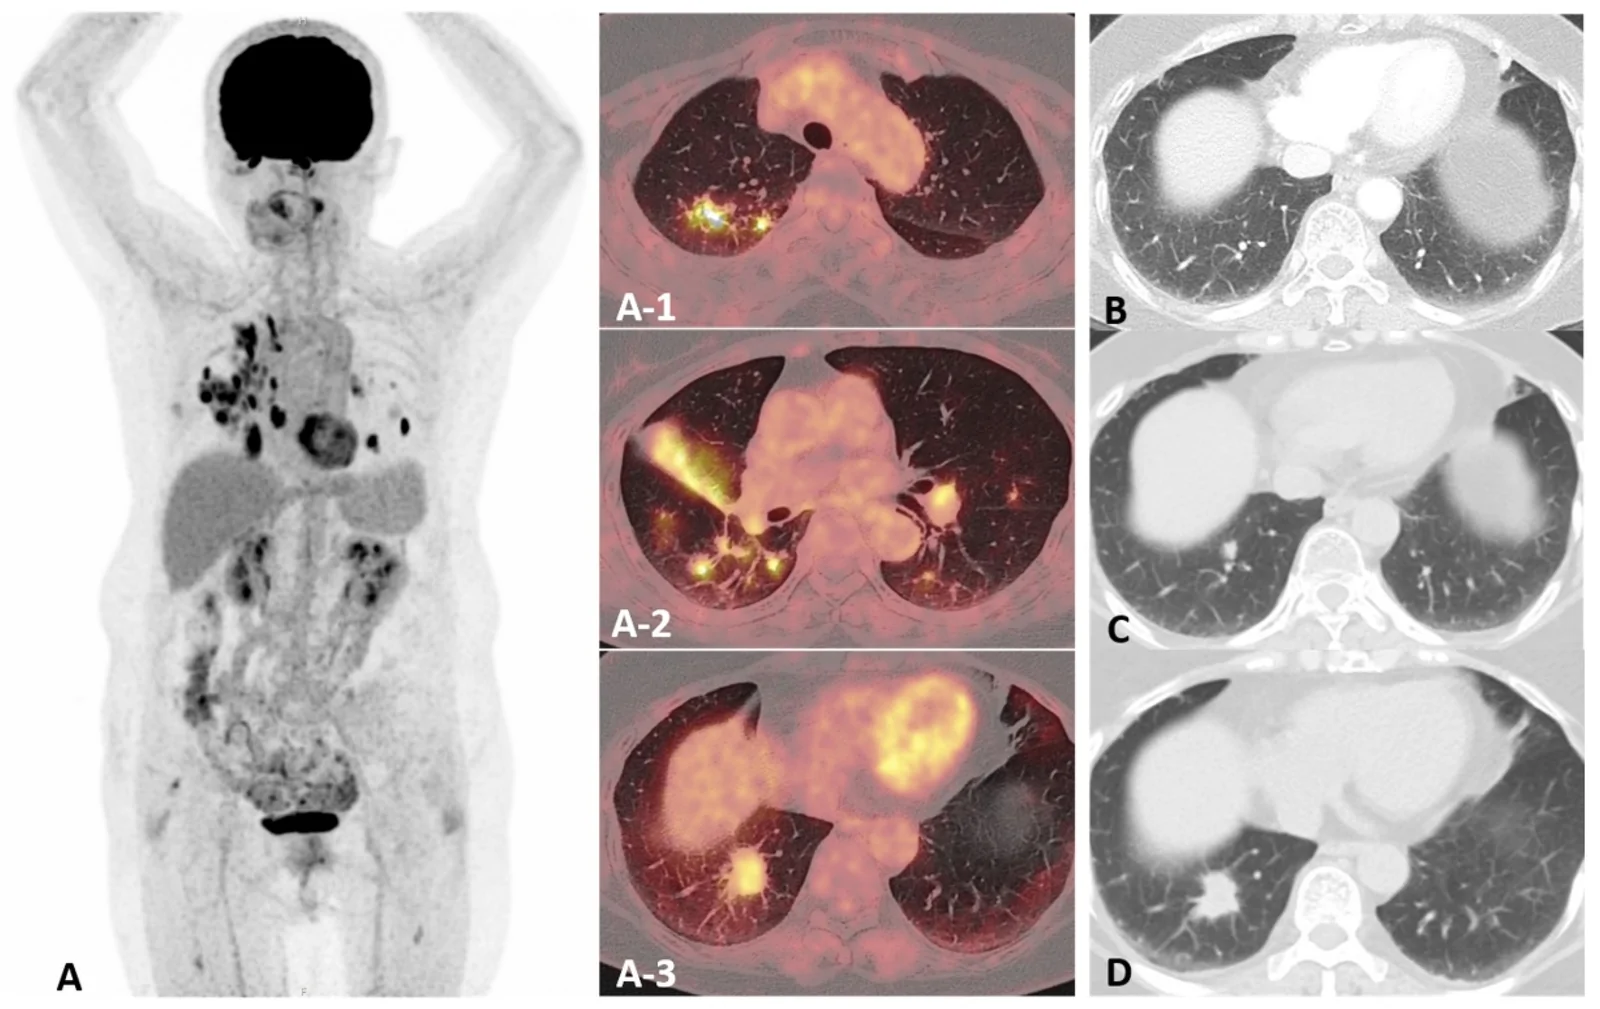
Metastatic cholangiocarcinoma coexisting with nontuberculous mycobacterial (NTM) infection. A woman with a history of common bile duct cancer 9 years earlier underwent FDG PET/CT (A,A-1–A-3) for multiple lung lesions. Prior CT scans obtained 4 years (B), 2 years (C), and 2 months (D) before the index FDG PET/CT were reviewed. The progressively enlarging right lower lobe mass was surgically resected and confirmed as adenocarcinoma, with clinicopathologic findings favoring pulmonary metastasis from the previous cholangiocarcinoma. The other resected lesions showed chronic granulomatous inflammation with multinucleated giant cells and necrosis, and tissue AFB culture grew nontuberculous mycobacteria, confirming NTM infection.
